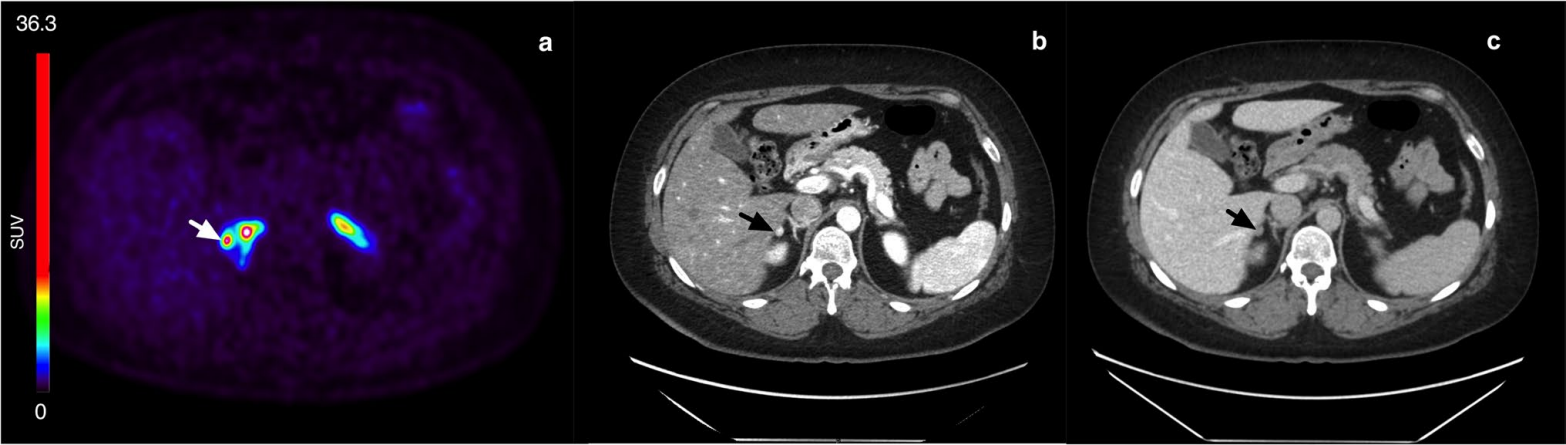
Right-sided Conn adenoma (arrow), patient 4. [^18^F]CETO PET 3 h post injection (a), arterial phase contrast-enhanced CT (b), and venous phase contrast-enhanced CT (c) where CT was performed 6 months before [^18^F]CETO PET
